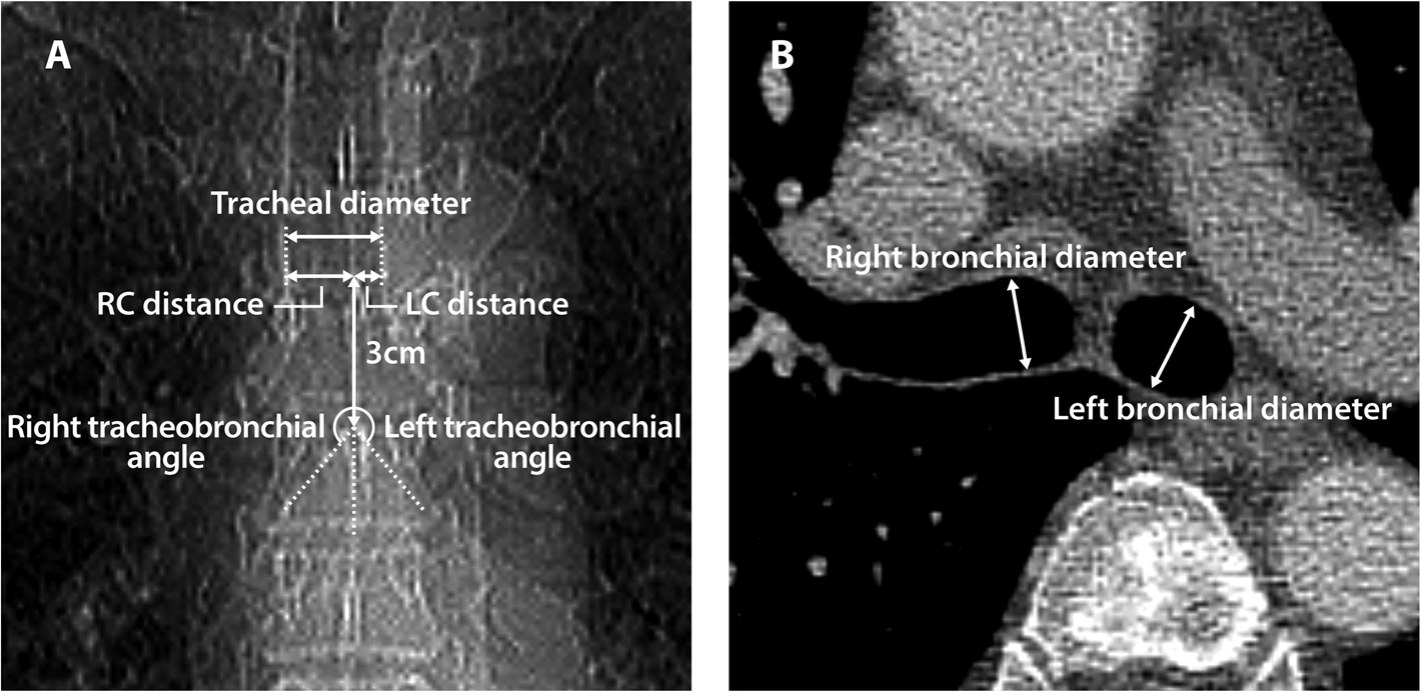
Anatomical variables that were measured on the plain chest radiography (a) and computed tomography (b)
